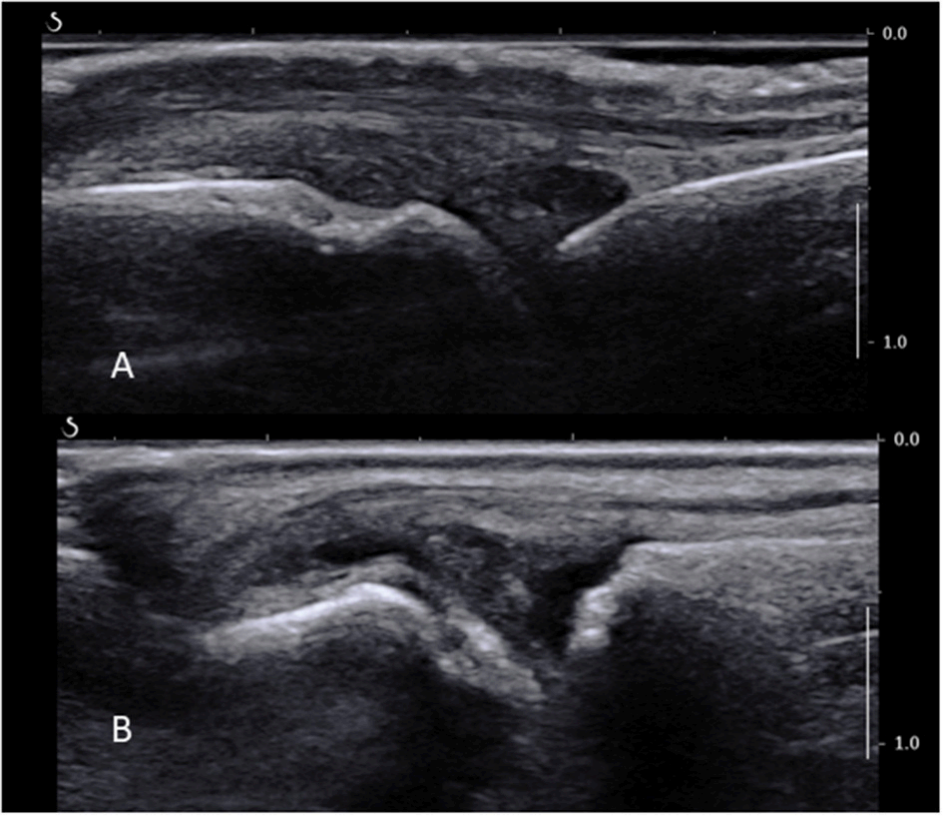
(A) Normal MCP joint on a longitudinal ultrasound view. Note the joint space, metacarpal head, proximal phalanx, and extensor tendon. (B) Long axis ultrasound scan of a fifth MTP joint showing synovial thickening proximally to the joint line overlying the metatarsal head, as well as a small amount of fluid.
